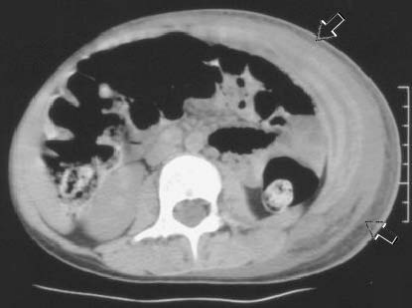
Abdominal wall edema. Unenhanced axial computed tomography scan through the mid-abdomen demonstrates extensive fluid tracking between abdominal wall muscle layers and coursing along anterior margins within subcutaneous fat (arrows).
